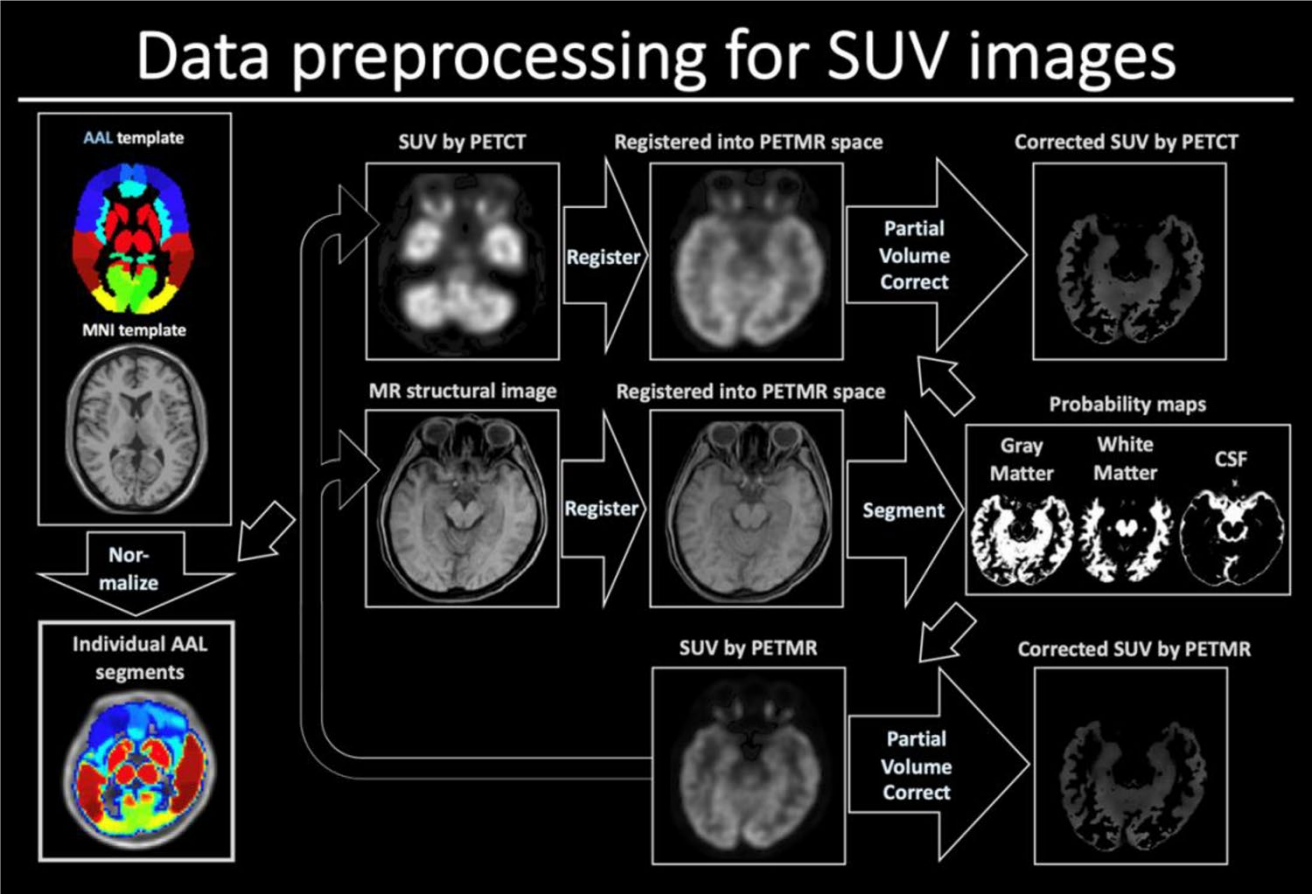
The image preprocessing for SUV images using PET/MR and PET/CT. All the individual PET/CT and structural images were registered into the orientation of individual PET/CT images. The structural images were then segmented into regions of gray matter (GM), white matter (WM), and CSF. The AAL3 template was normalized into the individual space. Then partial volume effect was corrected based on the segmented GM and WM maps for all the registered PET/CT and PET/MR images.
